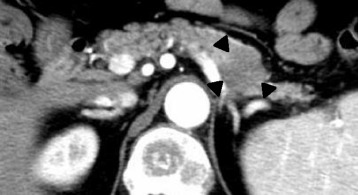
Contrast-enhanced whole-body CT image. A tumor of approximately 28 mm in diameter with poor enhancement was detected in the tail of the pancreas (*black arrowheads*)
